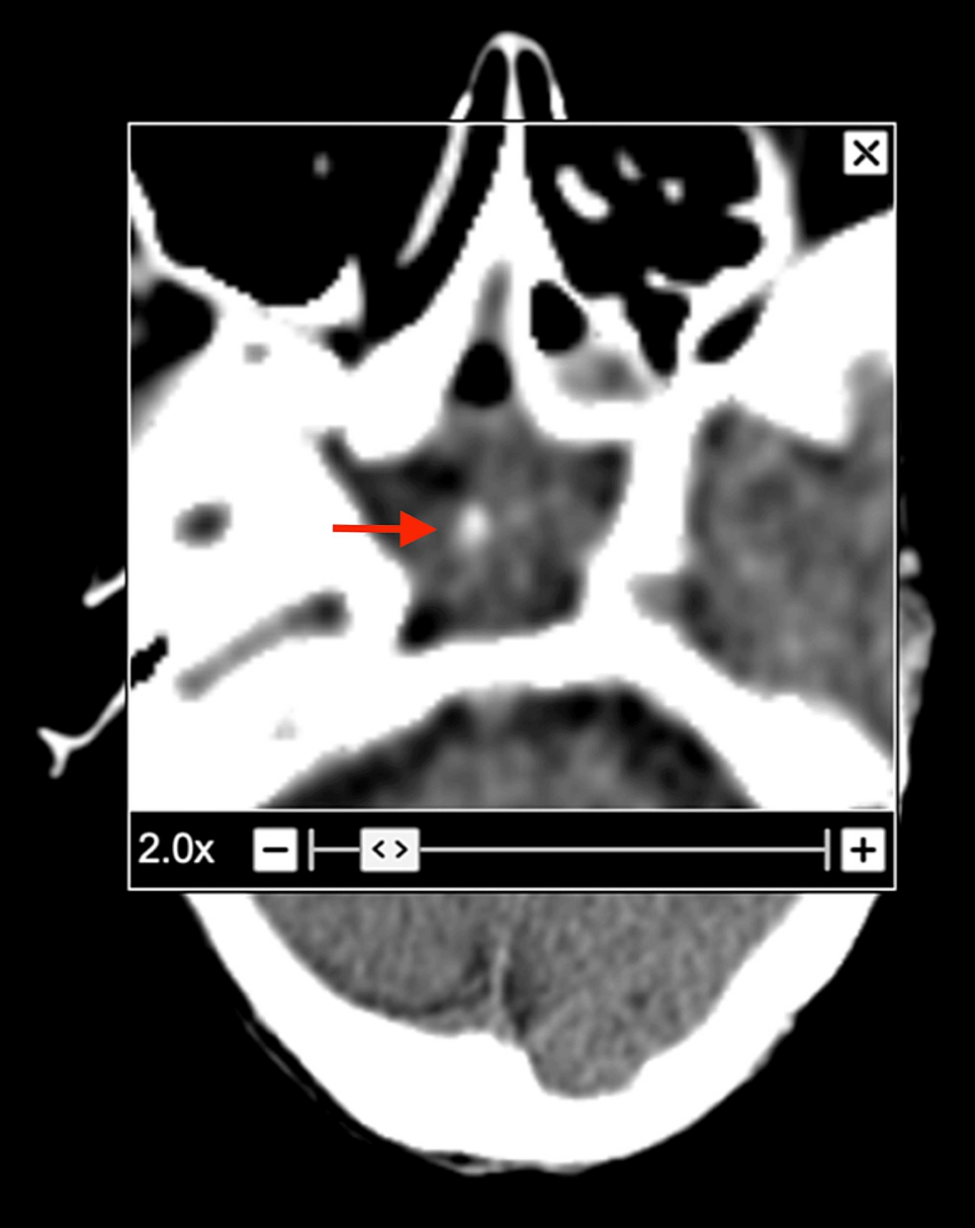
Head CT without contrast (axial view) with magnification showing calcification in the sphenoid sinus
